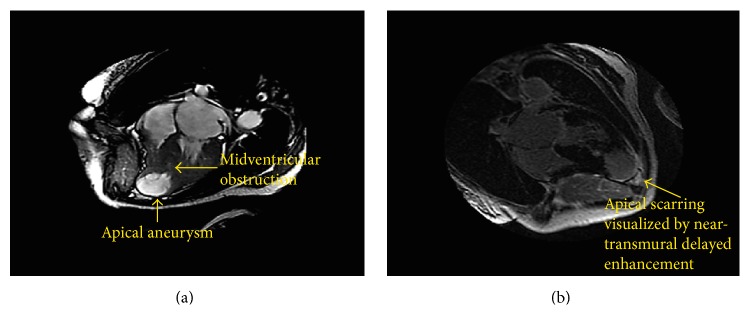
(a) Cine 4-chamber image of cardiac MRI done with steady state free precession imaging (SSFP) demonstrating diffuse hypertrophy with aneurysmal apex. (b) Delayed enhancement 4-chamber MRI image demonstrating thinned apex and apical scarring.
